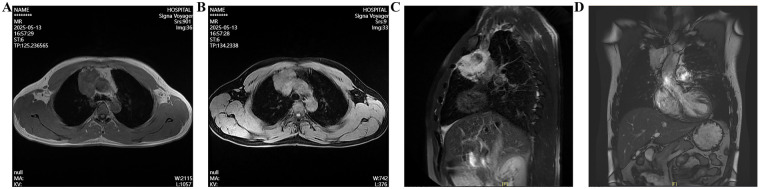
Chest magnetic resonance imaging shows a space-occupying lesion in the anterior mediastinum. (A–D) Phenomena of magnetic resonance at different periods and levels.
